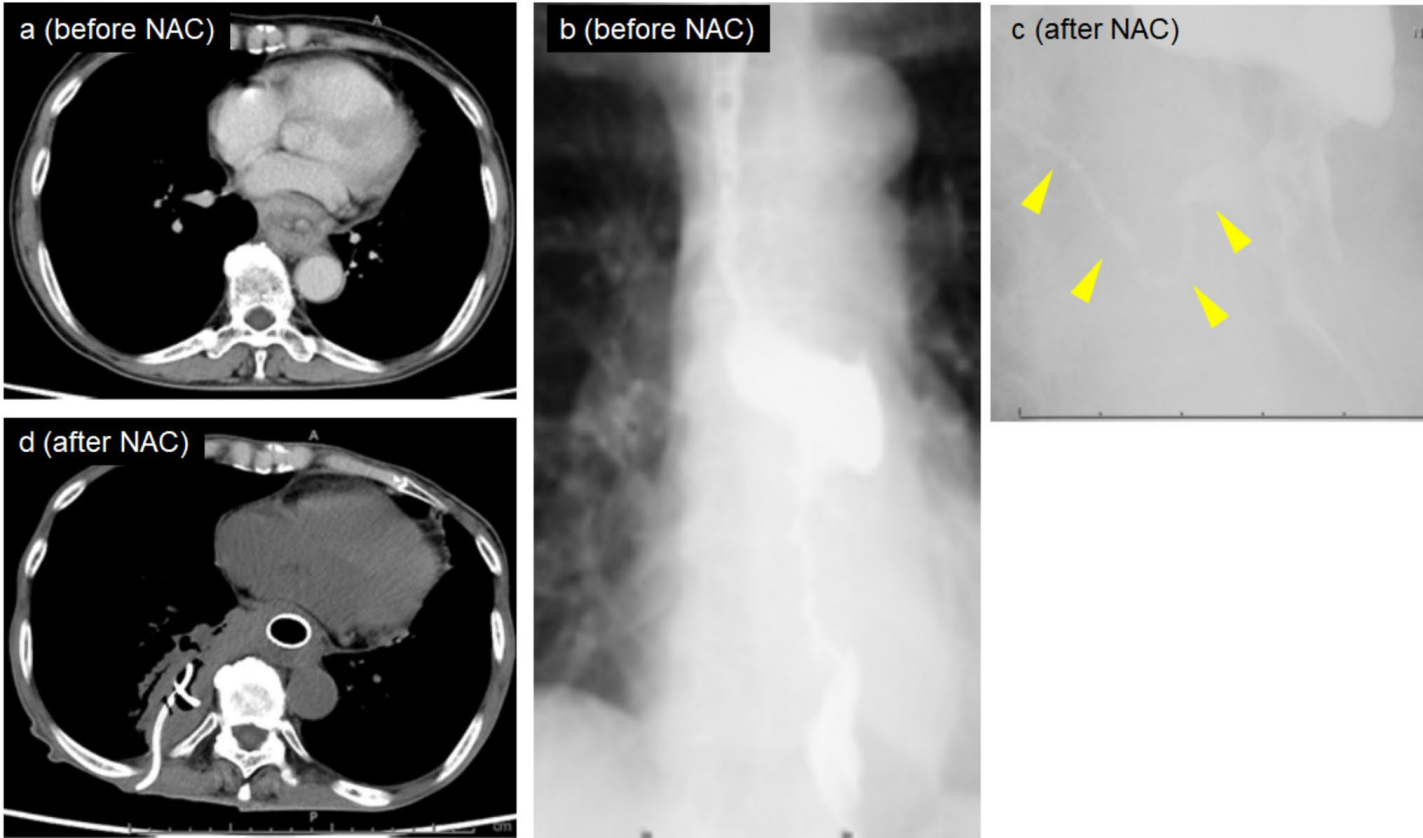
Clinical course images of Case 1. (a) Contrast-enhanced CT scan showing a tumor in the middle and lower thoracic esophagus. (b) Esophagography prior to DCF showing lower thoracic esophageal stenosis. (c) Esophagography after two cycles of DCF therapy showing persistent stenosis and an esophagorespiratory fistula (arrowhead). (d) CT image showing esophageal stent and right-sided pyothorax drainage. NAC: neoadjuvant chemotherapy
